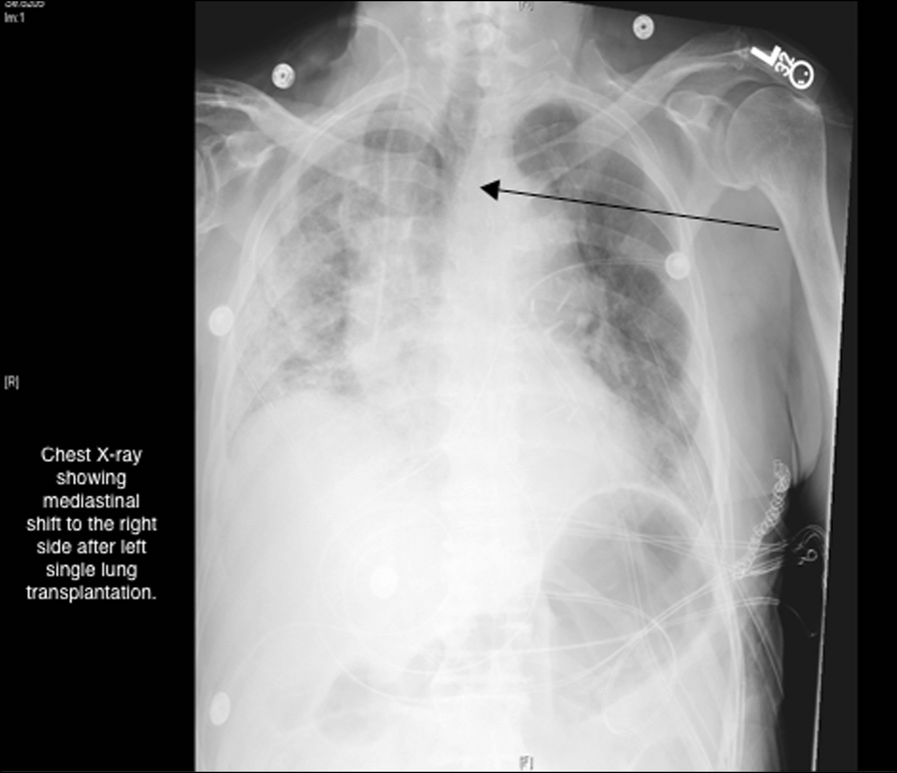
Post-Operative Chest X-ray Indicating a Mediastinal Shift. Arrow points to mediastinal shift to the right side after left lung transplantation
